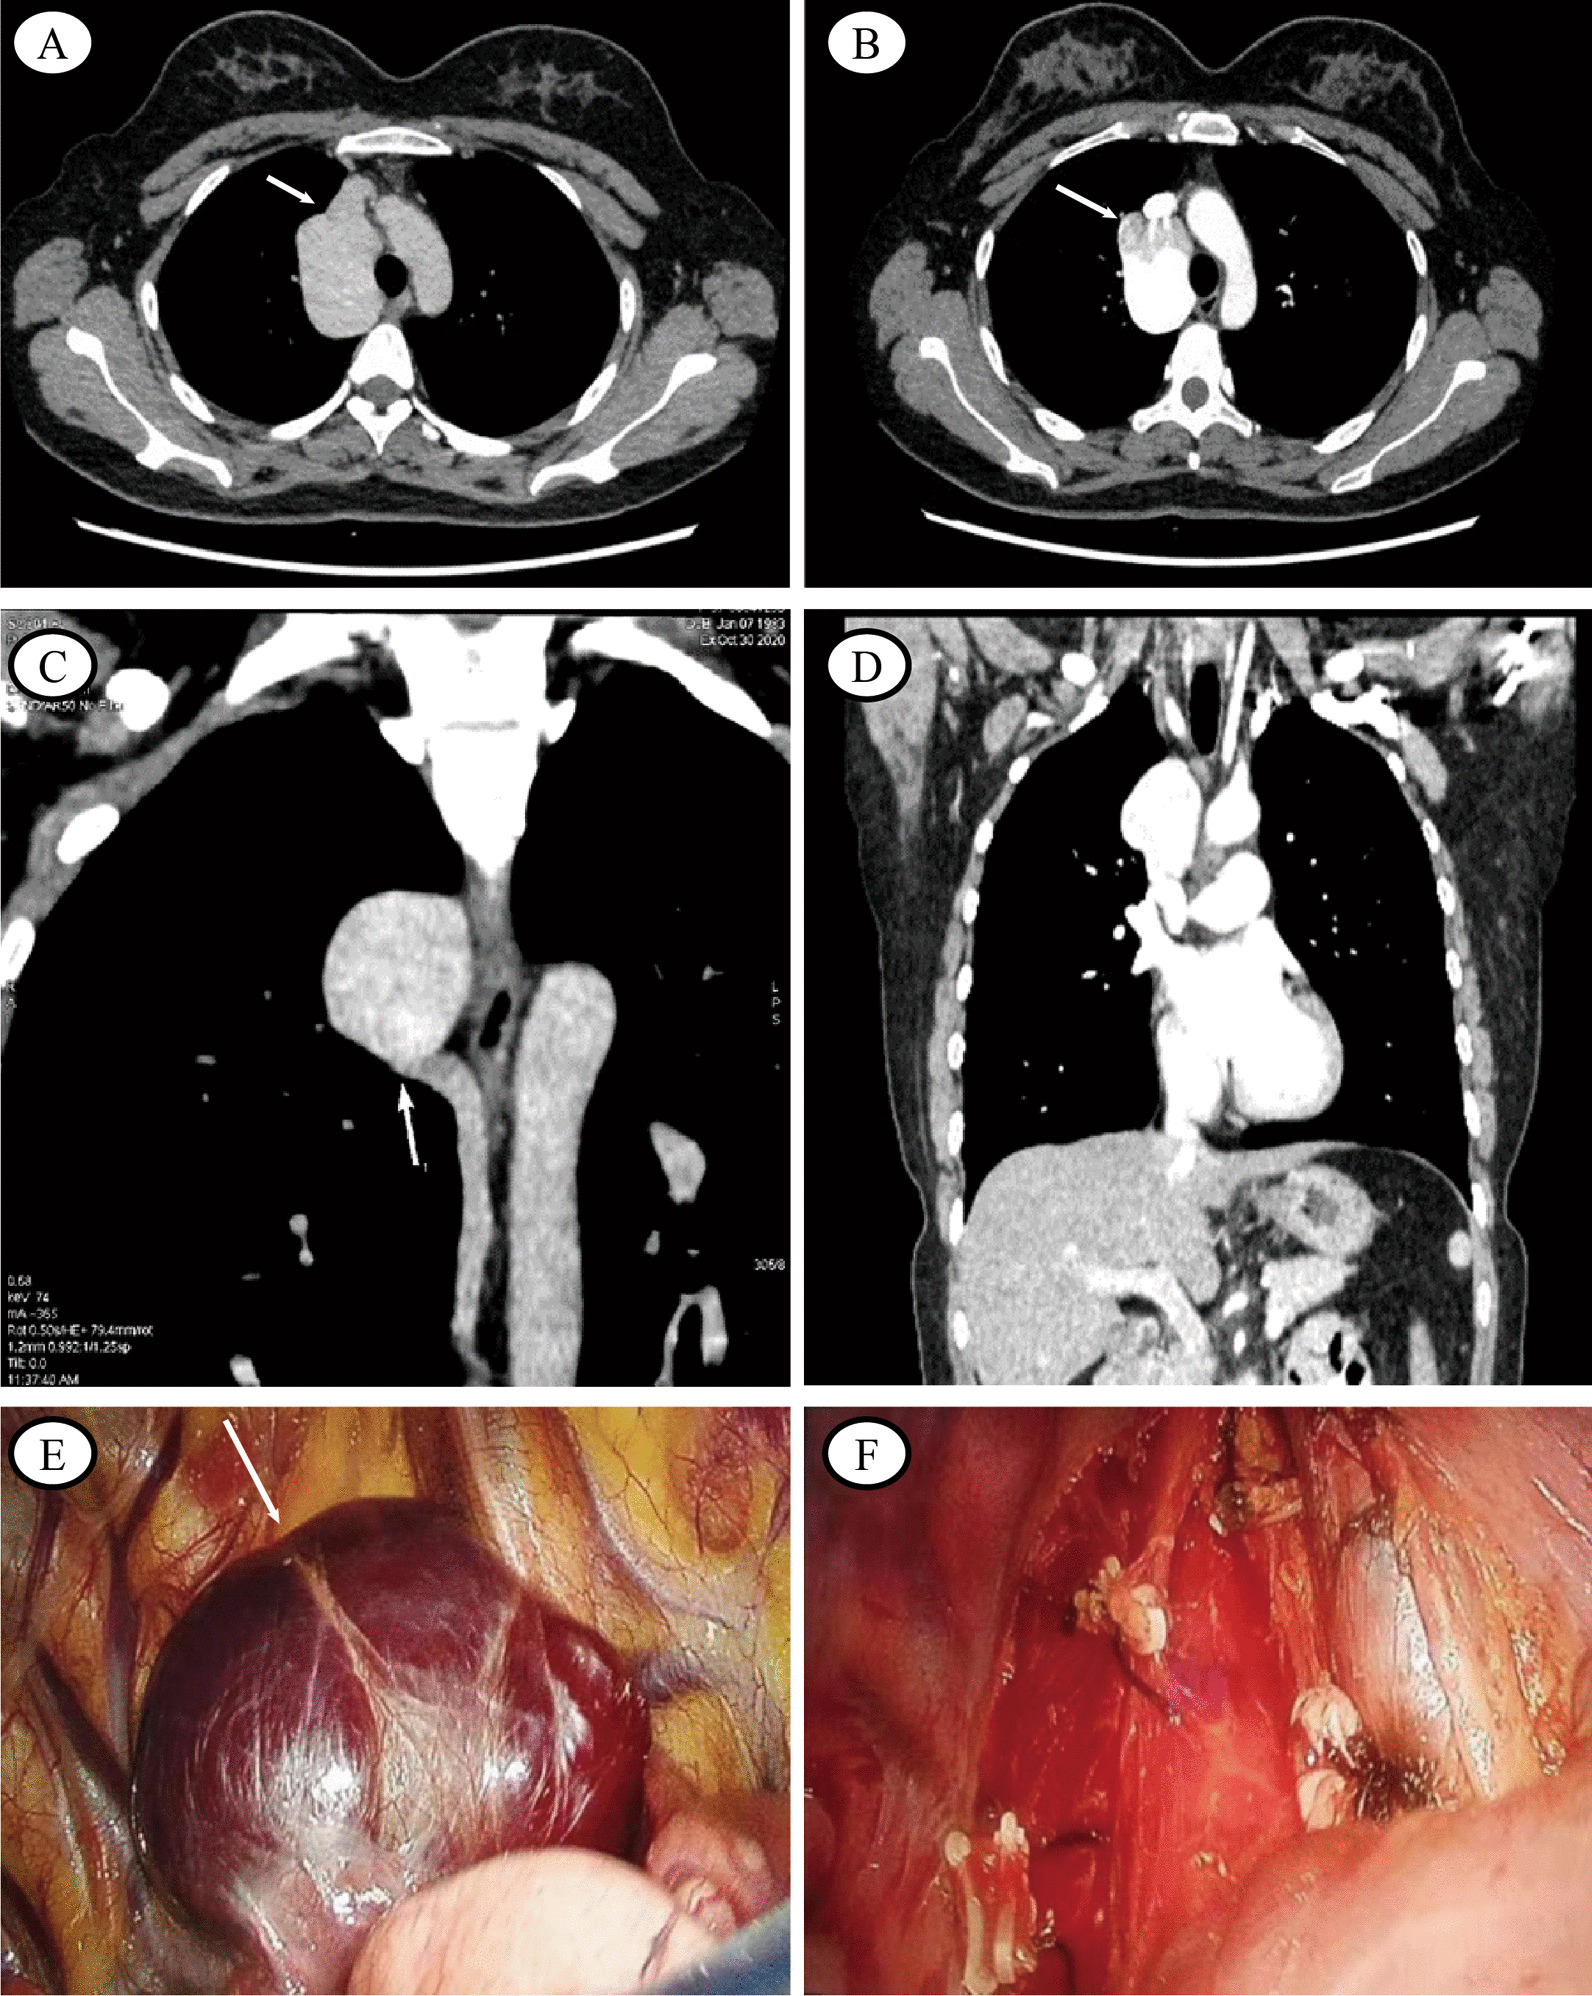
A, B: Chest computed tomography showed a soft-tissue mass in the right posterior mediastinum. Enhanced computed tomography scanning showed the mass with similar enhancement to the aortain(white arrow), and the internal density was uneven. C, D: Coronary reconstruction showed that the mass was connected with the azygos vein(white arrow). E: Thoracoscopic surgery revealed a mass arising from the azygos venous arch; there was light adhesion. F: The venomous mass was completely removed
